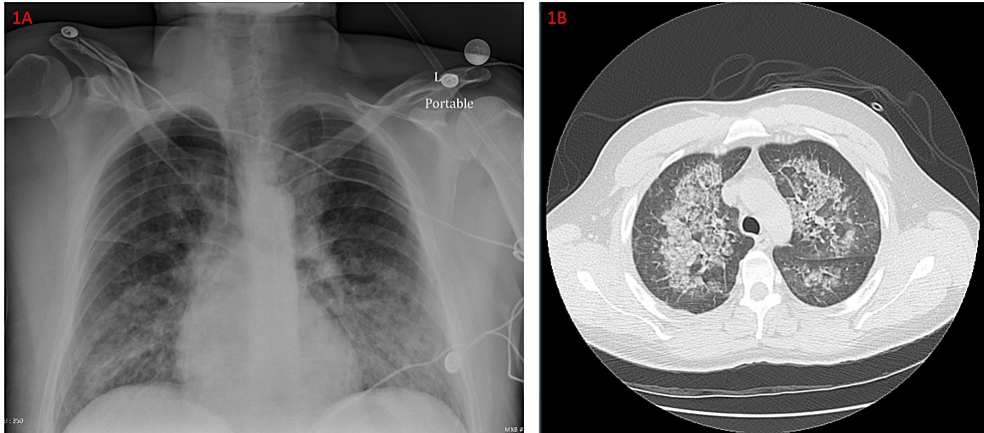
A chest radiograph on admission shows diffuse bilateral interstitial opacities (1A). The patient’s CT chest on admission shows diffuse bilateral ground-glass opacities (1B).
